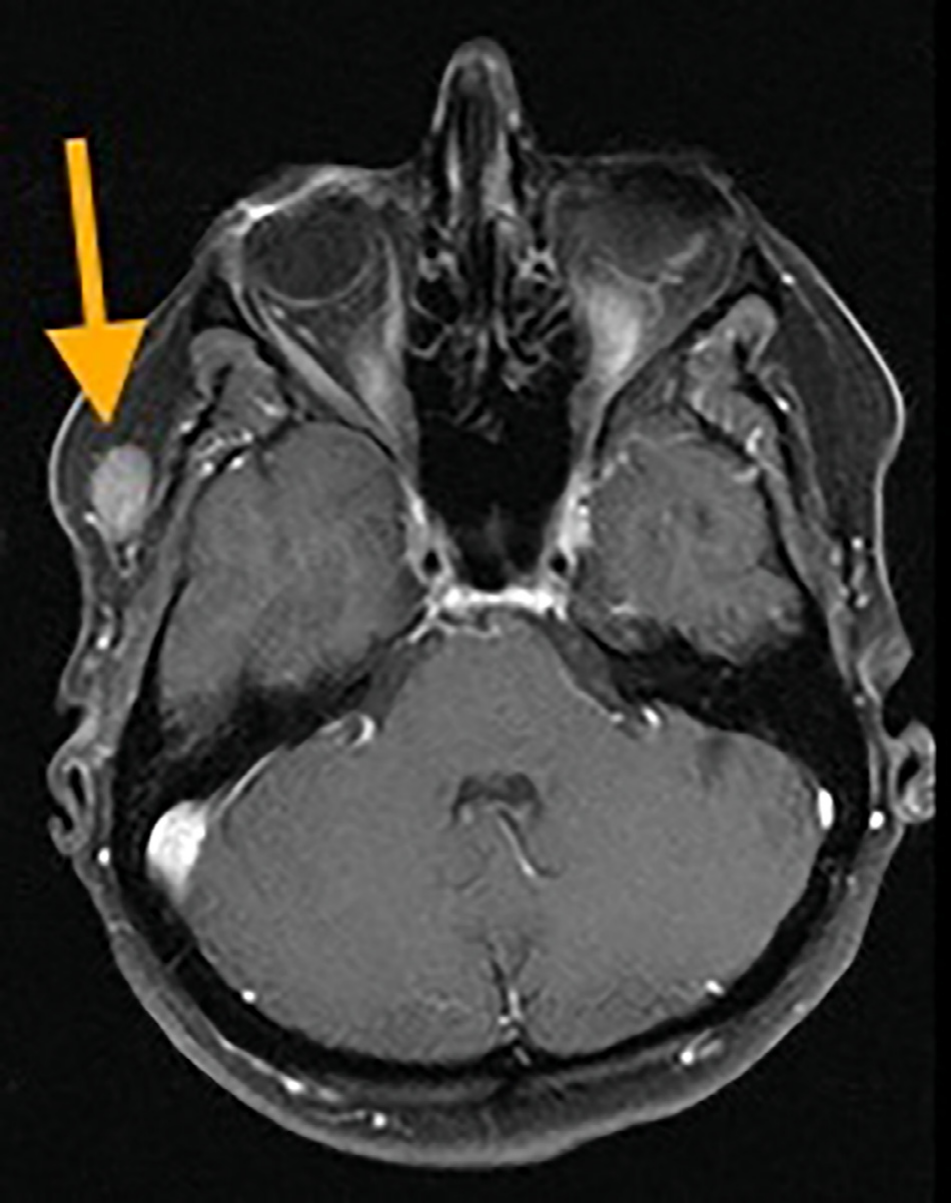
T1-weighted fat-saturated post-contrast MRI sequence demonstrating a 1.6 cm enhancing lesion in the right temporal subcutaneous tissues (arrow). There is normal enhancement of the right sigmoid sinus. No intracranial lesion was identified.
